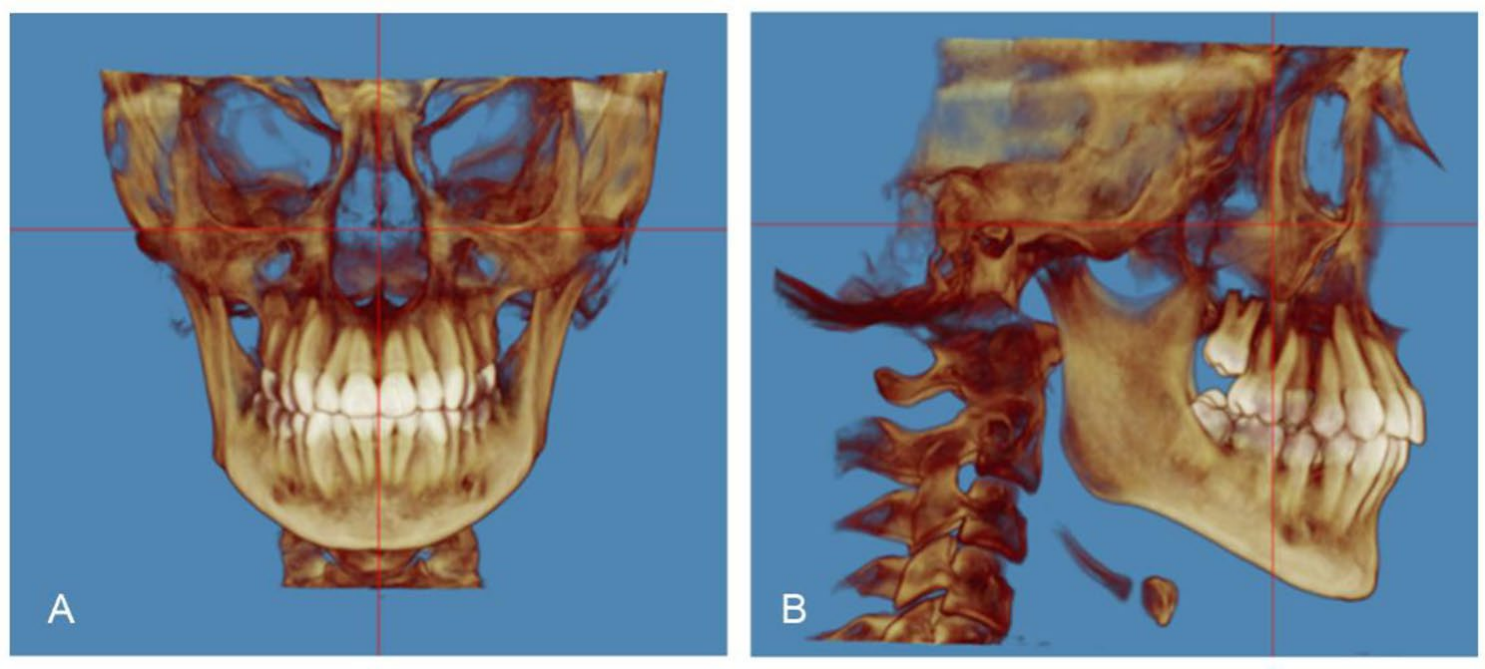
CBCT image orientations (A) Midsagittal plane (B) Axial and coronal plane
